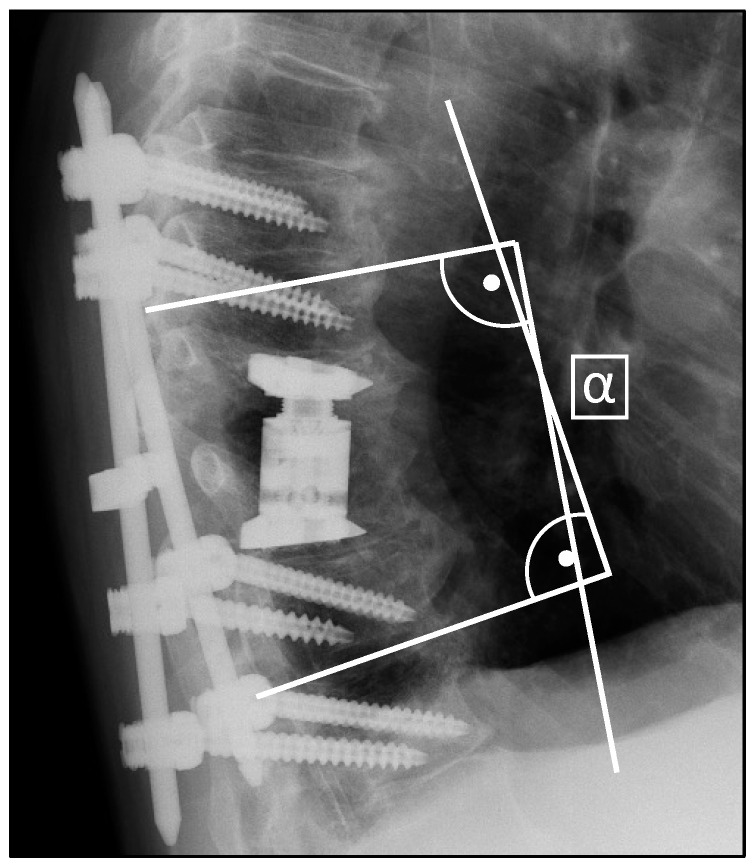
Measurement of the bi-segmental kyphotic endplate angle (BKA) in medio-lateral X-rays.
